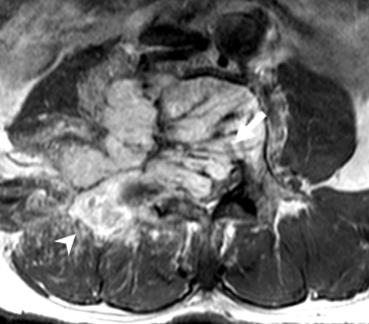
Pre-percutaneous embolization. Axial *T*_1_ weighted contrast-enhanced MRI of the lumbar spine showing the haemangioblastoma. Significant intraspinal tumour extension is present (solid arrow). An avidly enhancing extradural component is also noted at the right posterior aspect of the tumour (arrowhead).
